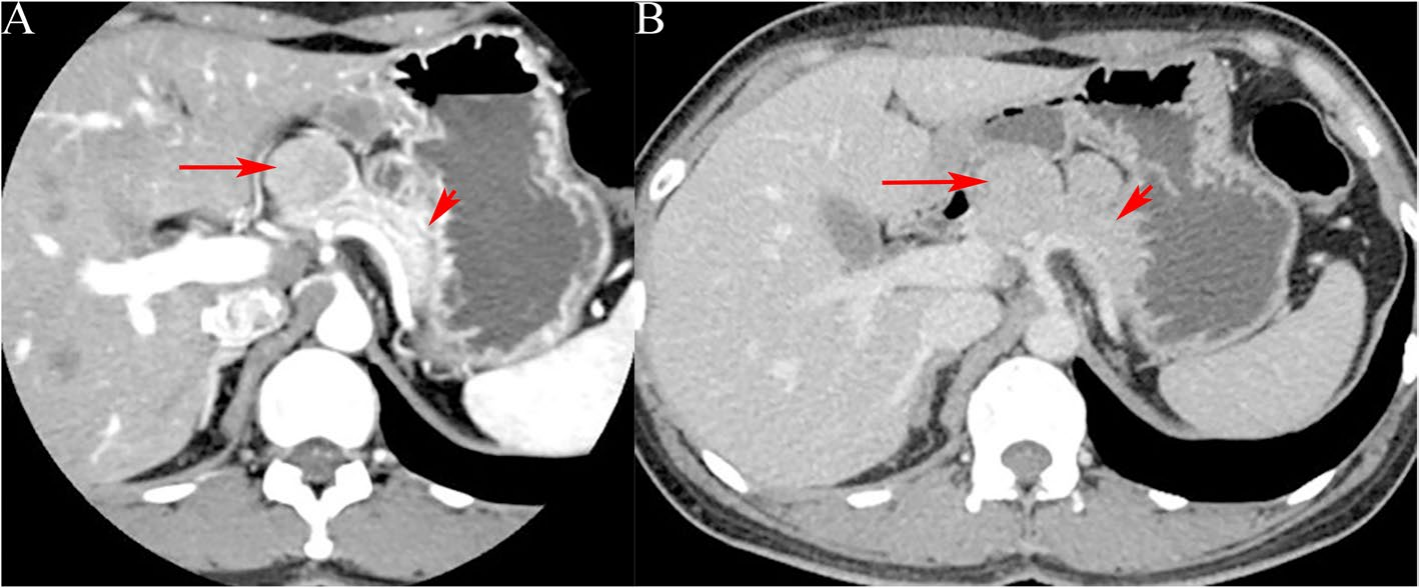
A 47-year-old female with solid pseudopapillary tumor. a CT target scanning shows that the SPTP is located on the pancreatic neck with a complete capsule and solid tumors, and the tumors (long arrow) are enhanced to a lower degree than the normal pancreatic parenchyma (short arrow) during the pancreatic parenchymal phase; b the tumors (long arrow) are enhanced to the same degree as the normal pancreatic parenchyma (short arrow) during the delayed phase
